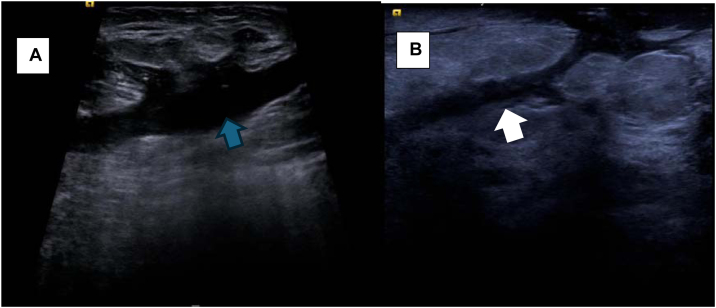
Ultrasound findings of idiopathic granulomatous mastitis – (A) Collection with anechoic content and some suspended debris (blue arrow), located in the inferolateral quadrant of the left breast; (B) Hypoechoic collection, located in the right breast, with a periareolar fistulous tract (white arrow).
